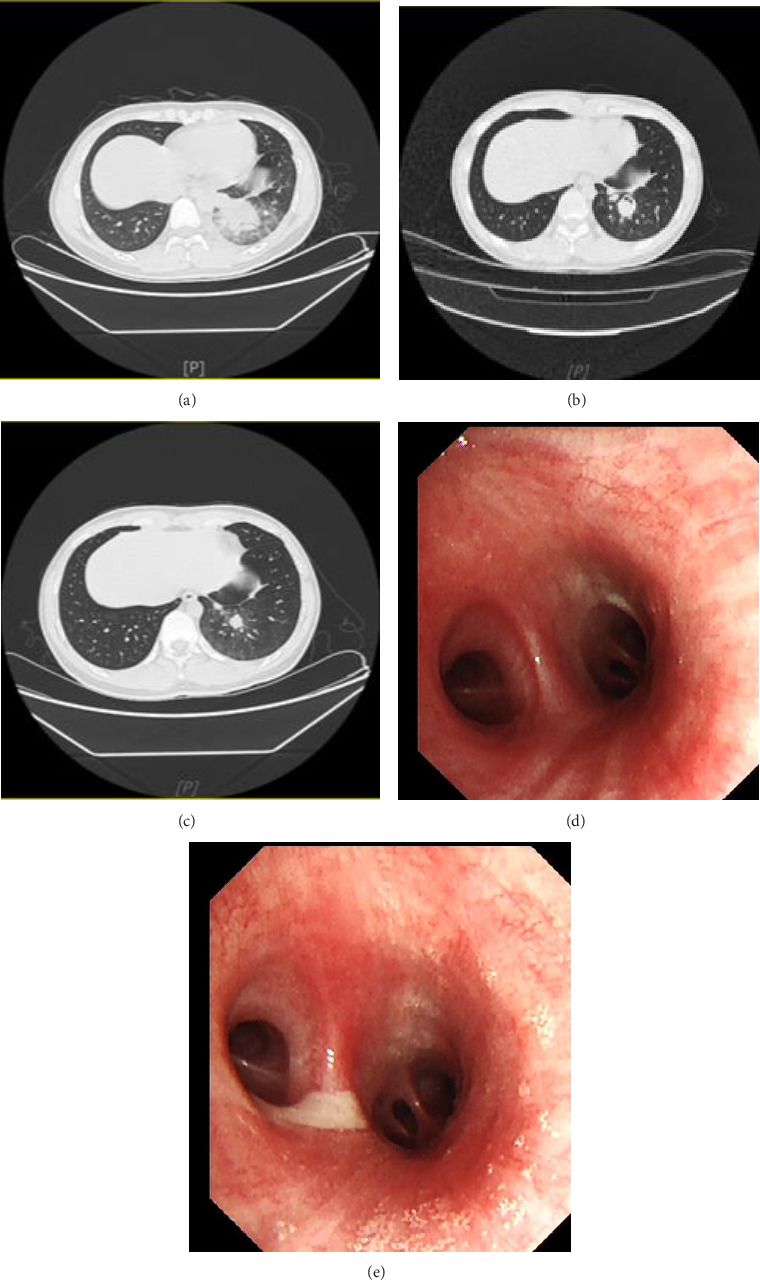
(a) July 17, 2024 chest CT showed massed solid and patchy shadows in the left lower lobe, limited thickened adhesions of the left pleura with pleural effusion. (b) July 23, 2024 chest CT showed multiple round-like hypodense shadows in the lower lobe of the left lung, and lung abscess formation was considered. The extent of the infection and the left pleural effusion were reduced compared to the previous CT. (c) July 29, 2024 chest CT showed significant resorption of a lesion in the lower lobe of the left lung compared to the previous CT. (d) July 22, 2024 bronchoscopy revealed reduced congestion and edema of the left lower lobe bronchial mucosa and disappearance of purulent secretions. (e) July 18, 2024 bronchoscopy revealed congestion and edema of the left lower lobe bronchial mucosa with purulent secretions.
